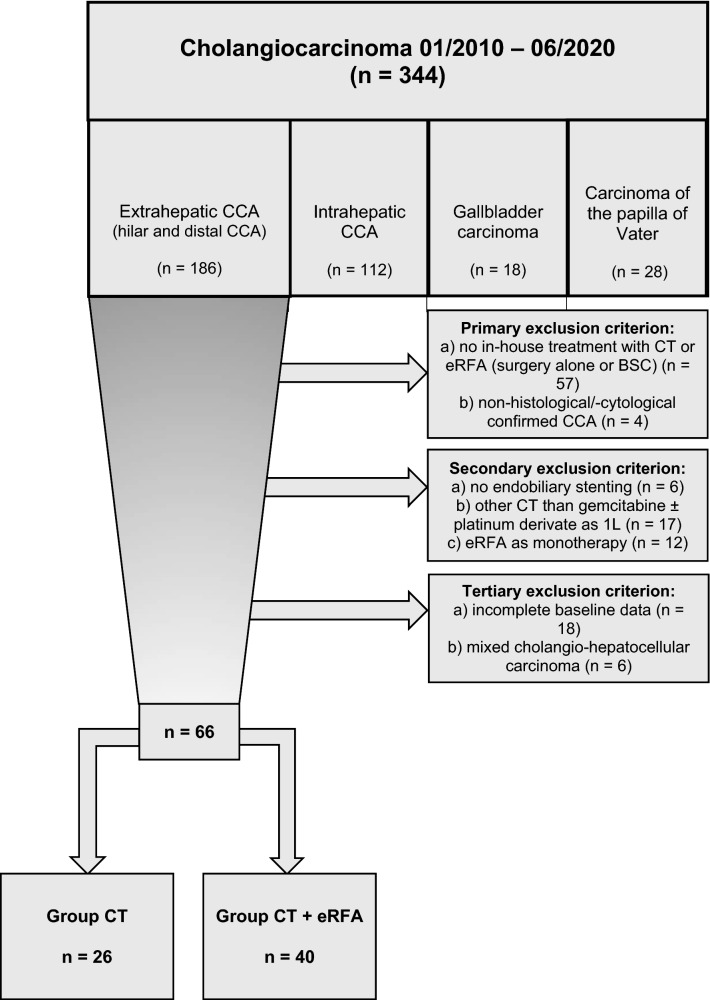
Flow-chart of patients. *1L* first-line, *BSC* best supportive care, *CCA* cholangiocarcinoma, *CT* chemotherapy, *eRFA* endobiliary radiofrequency ablation, *n* number.
